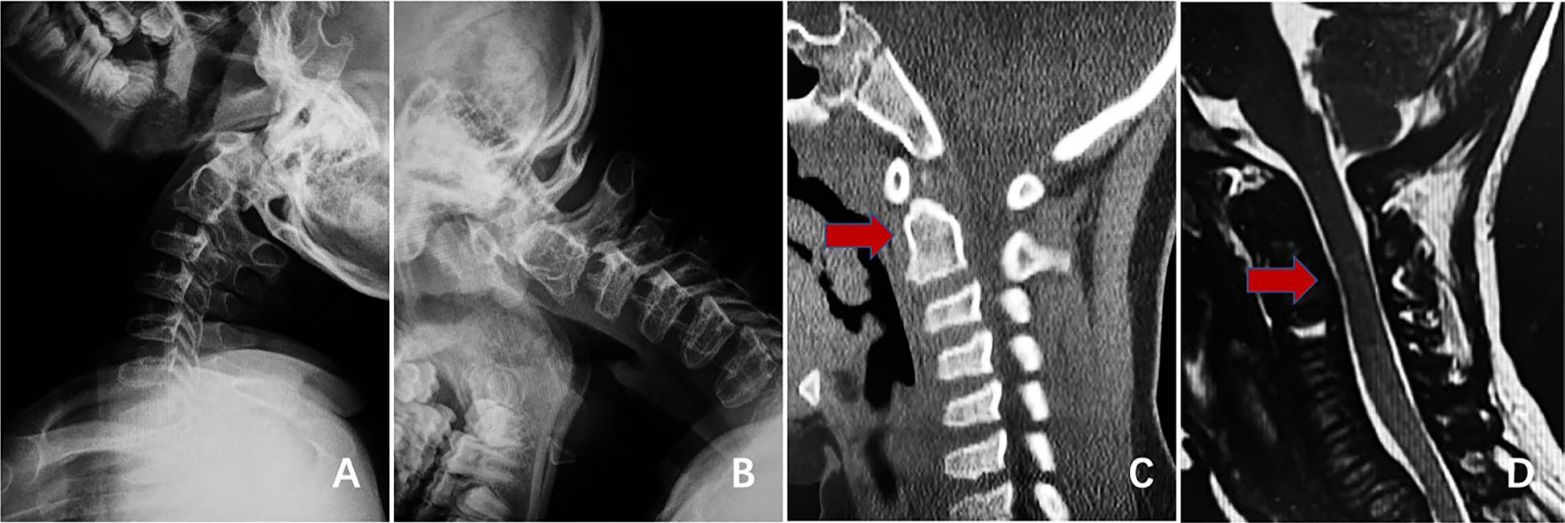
Imaging findings of a 10-year-old patient who presented to our outpatient department with a swaying gait while walking. Dynamic radiograph showing no significant atlantoaxial dislocation or instability (A, B). CT showing odontoid dysplasia. The patient is currently under follow-up observation, as there are no obvious signs of spinal stenosis or spinal cord compression (C, D)
